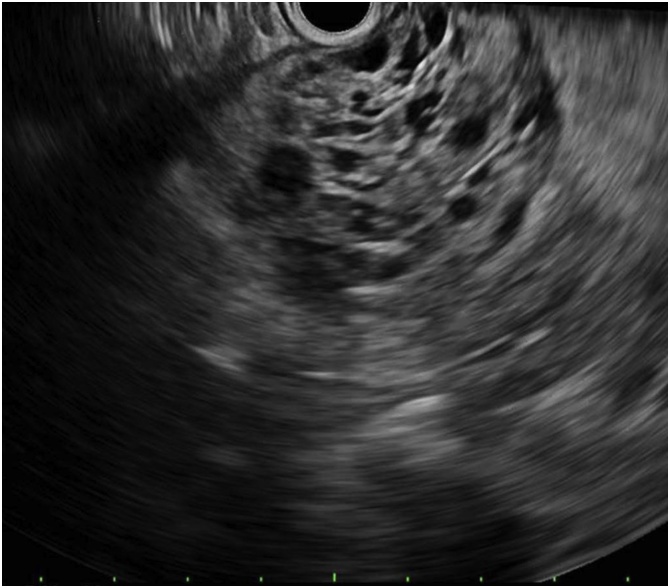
Endoscopic ultrasound. An anechoic, multicystic, septated and shadowing lesion suggestive of a cyst was identified in the pancreatic body and in the pancreatic tail. No communication with the pancreatic duct. Many thinly septated compartments, with no associated mass or internal debris within the fluid-filled cavity.
